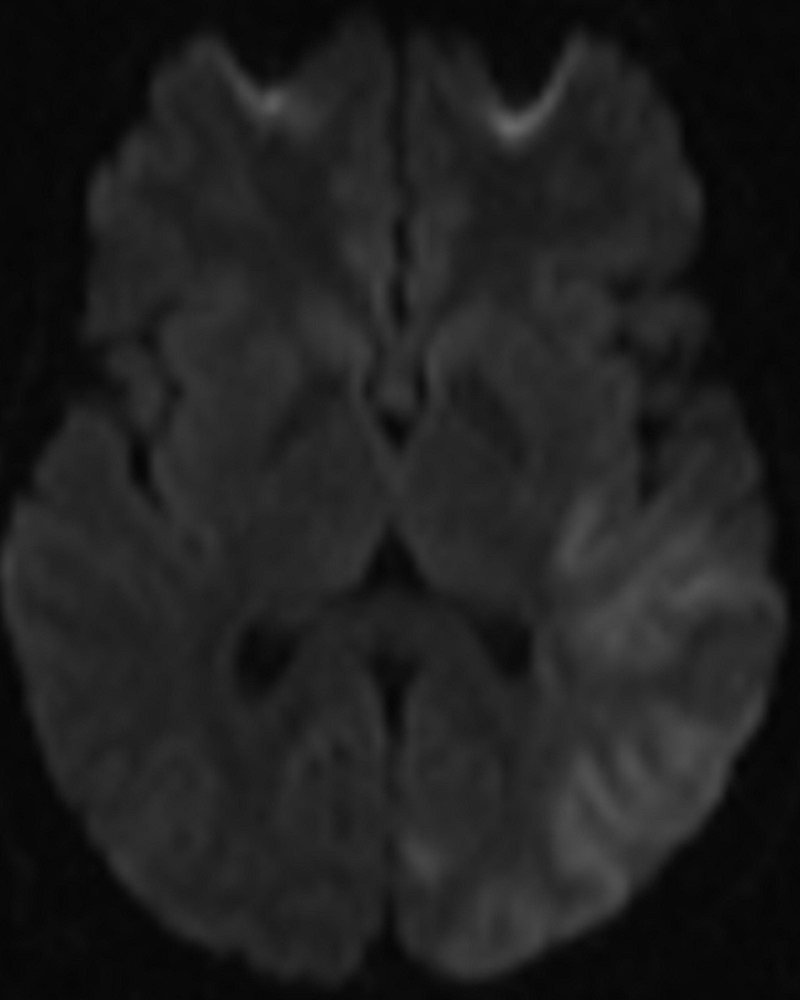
Diffusion MRI of the brain The MRI diffusion sequence shows hyperintensity of the left temporal lobe which is compatible with an acute stroke.
